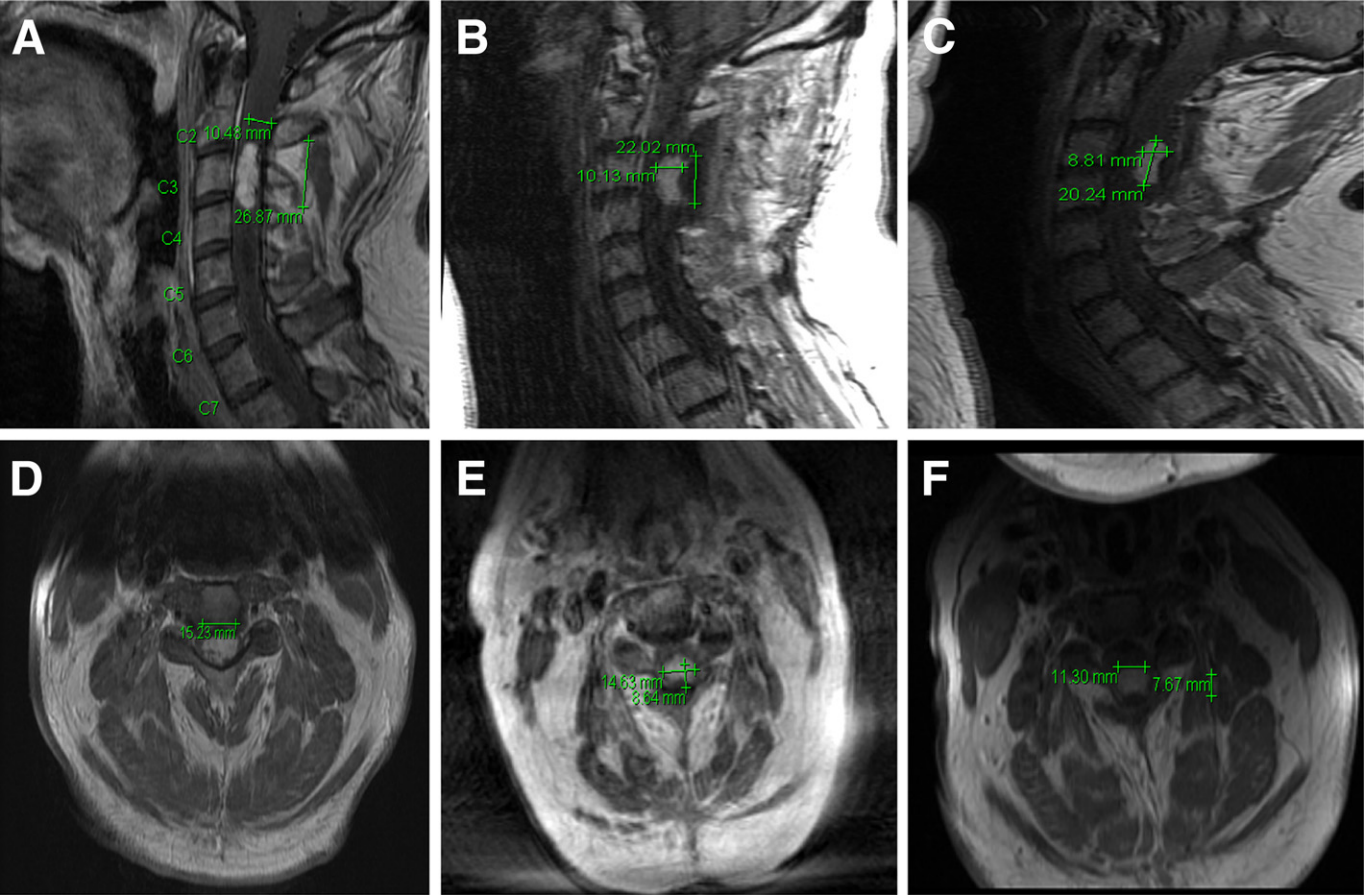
Bevacizumab resulted in tumor regression of a cervical cord hemangioblastoma. This is a sagittal and axial magnetic resonance imaging view of the cervical spine, post gadolinium-diethylene triamine pentaacetic acid administration. Figure 1A (sagittal) and 1D (axial) demonstrate the pre-bevacizumab baseline scan. Figure 1B (sagittal) and 1E (axial) demonstrate tumor regression following two cycles of bevacizumab. Figure 1C (sagittal) and 1F (axial) demonstrate further reduction in the enhancing tumor following an additional four cycles of bevacizumab.
